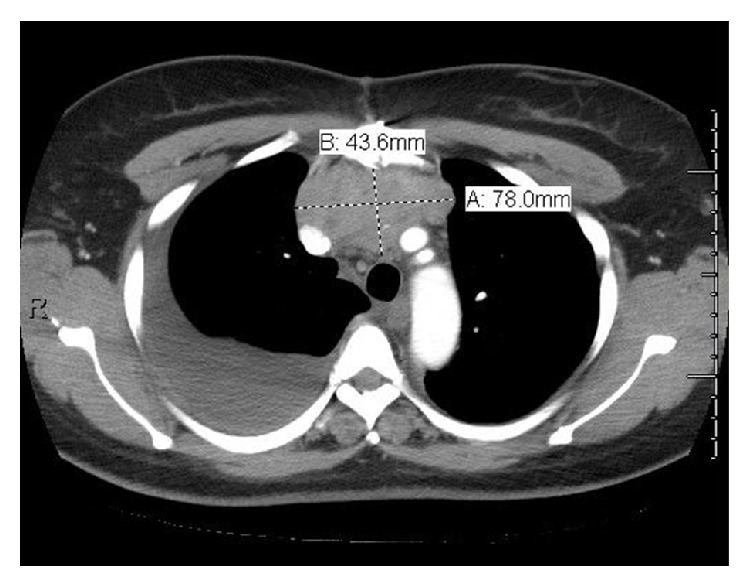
CT scan of the chest after chemotherapy with reduction in size of the tumor.
